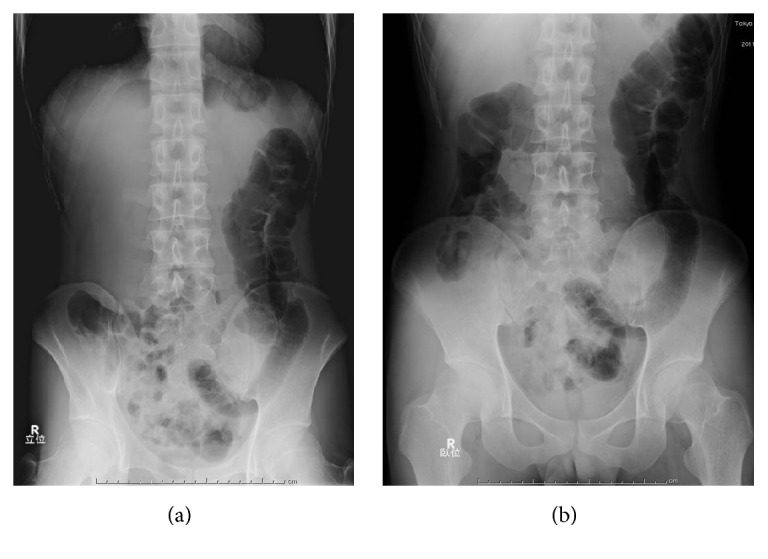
(a) Plain abdominal radiograph, upright position. (b) Plain abdominal radiograph, supine position. Images of gas in the left side of the colon in upright and supine positions are nearly identical.
